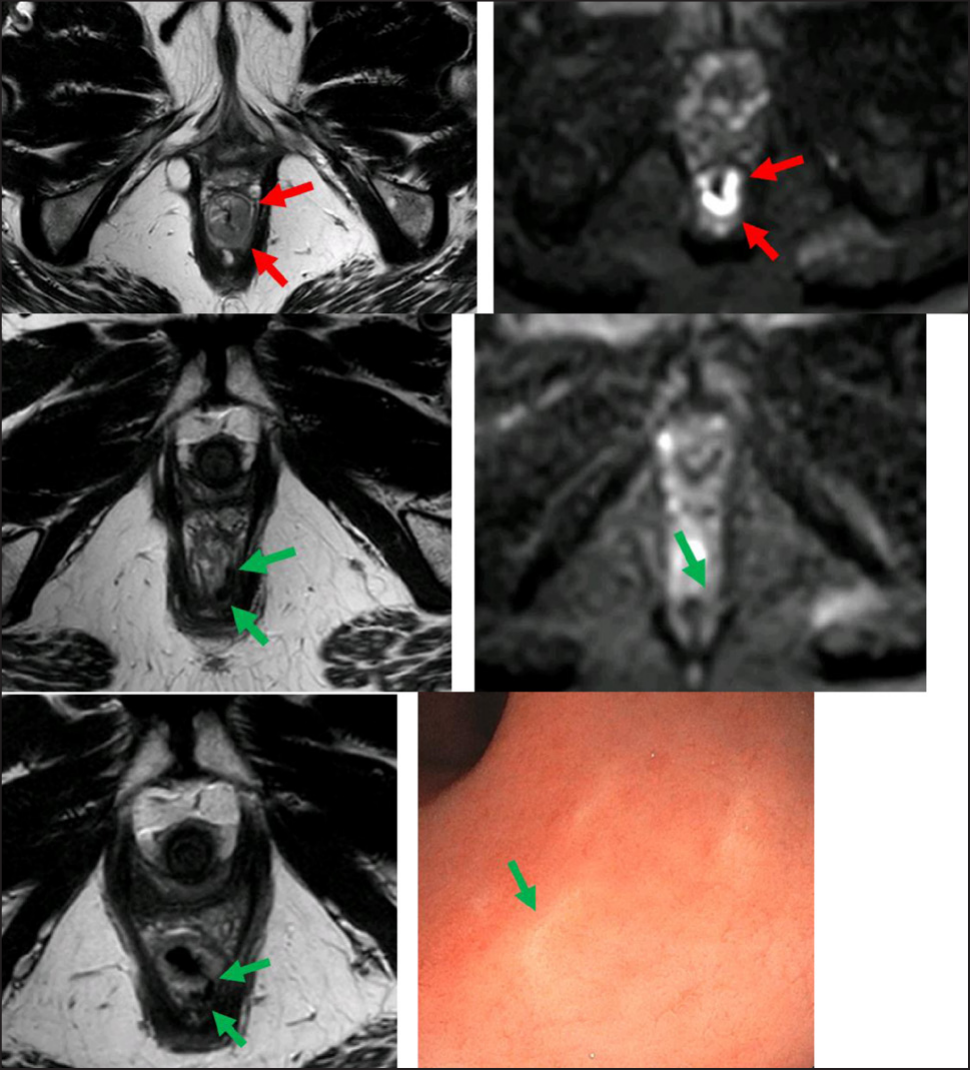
Patient with a tumor at the left posterolateral side of the distal rectum (see arrows on the images in the top row). After radiochemotherapy, the original site of the tumor has become very hypo-intense on the T2-weighted images with no restricted diffusion (see arrows on the ilages in the middle row), reflecting a complete response. This was confirmed by endoscopy, which showed a white scar (see arrow on the right bottom image).
